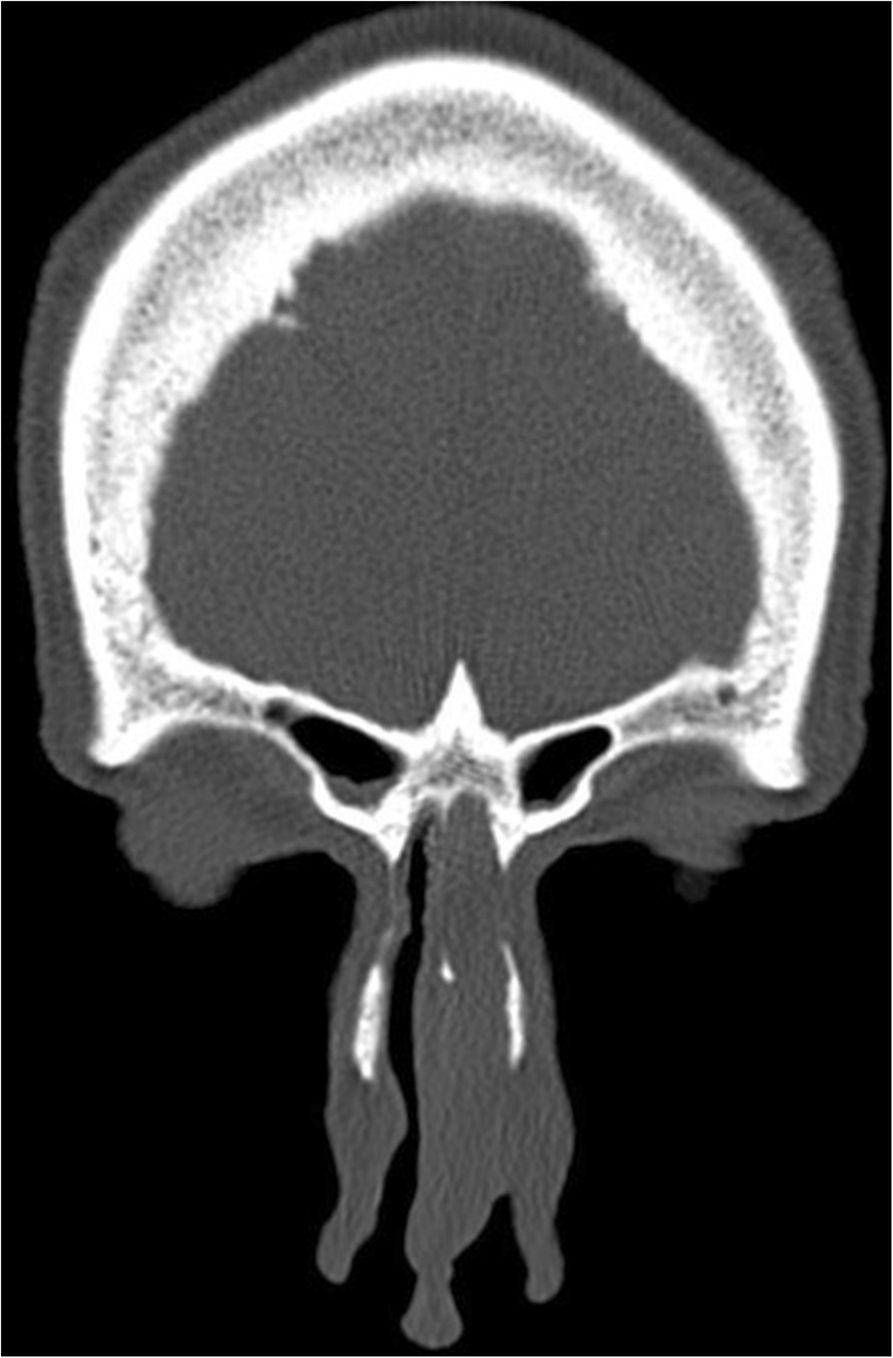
Coronal view on computed tomography showing a well-circumscribed septal mass.
